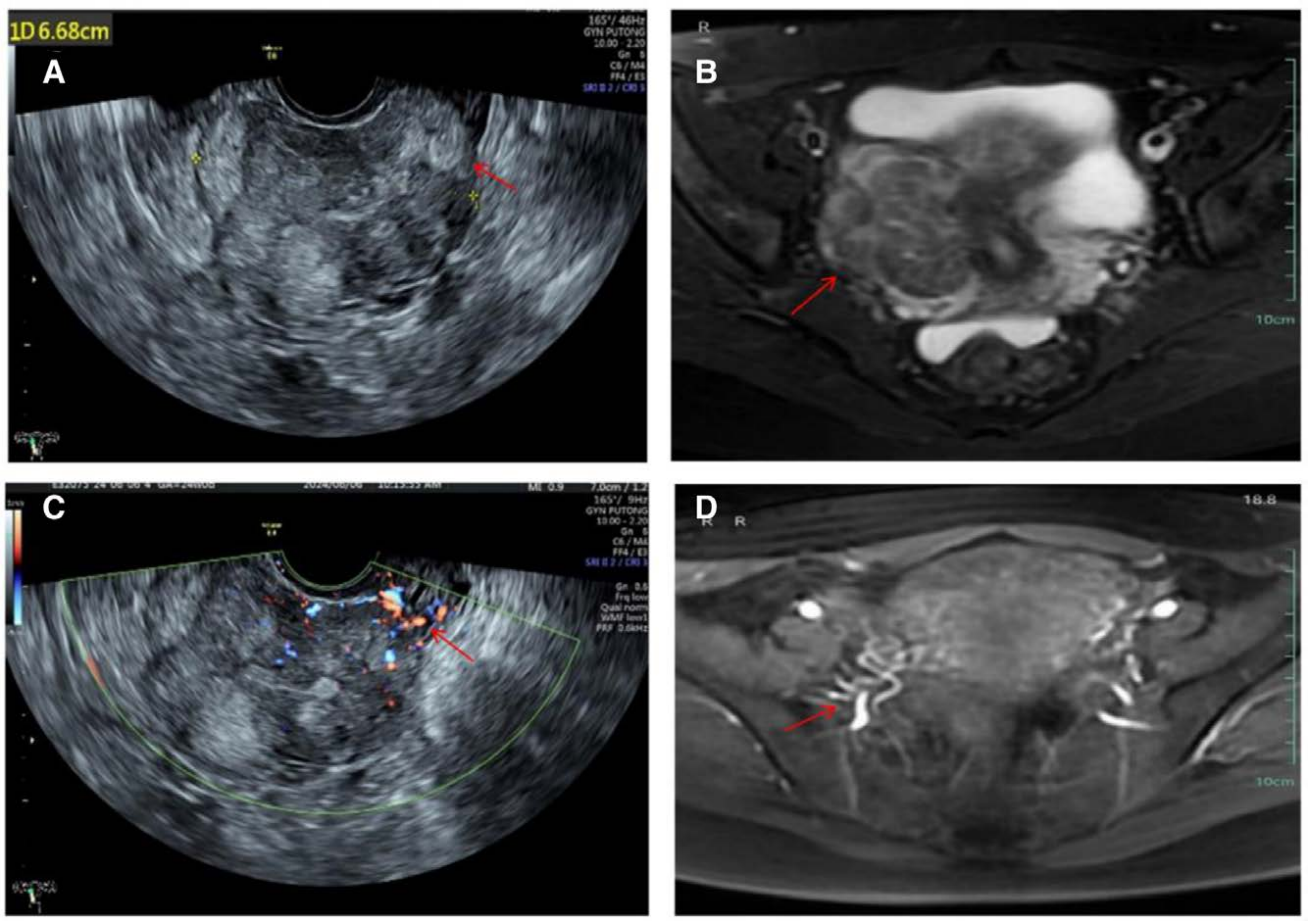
The clinical and pathological features of Patient 1. (A and B) Pelvic ultrasonography and MRI shows the location and morphology of the tumor (approximately 6 cm in diameter). (C and D) CDFI shows a rich blood supply with visible vessel penetration within the mass. The contrast agent shows thickening of the contrast-enhanced blood vessels. CDFI = color Doppler flow imaging, MRI = magnetic resonance imaging.
